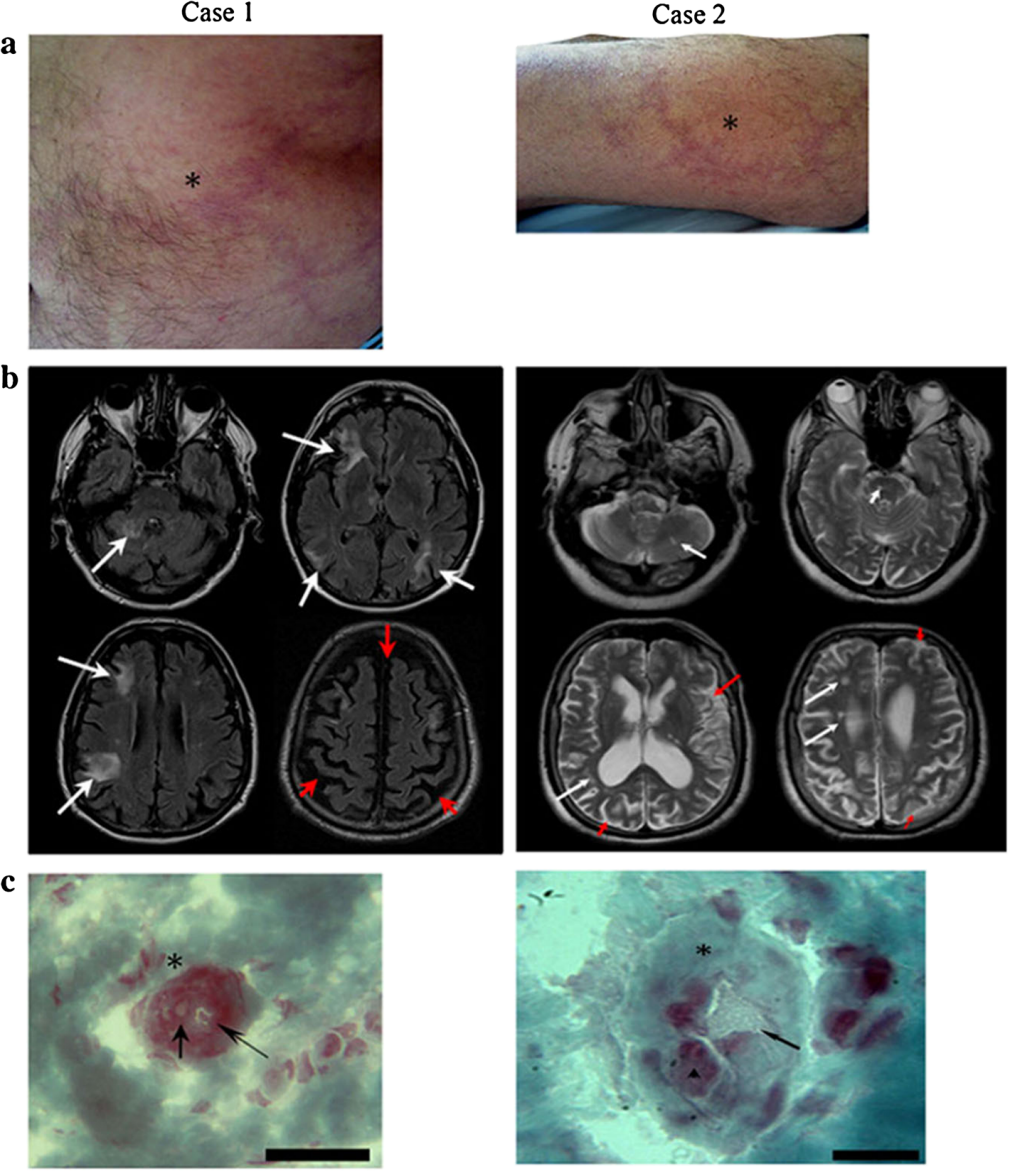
(two cases of SS ) a, diffused livedo racemosa (asterisks); b, FLAIR/T2 MRI brain scan showing multiple ischemic lesions (white arrows) and cortical atrophy (red arrows); c, skin biopsy showed intra-capillary (thin arrow) and parietal (thick arrow) widespread thrombosis; scale bar 100μm (Case1) and 50 μm (Case2). (courtesy of Dr. Alessandro Tessitore).
